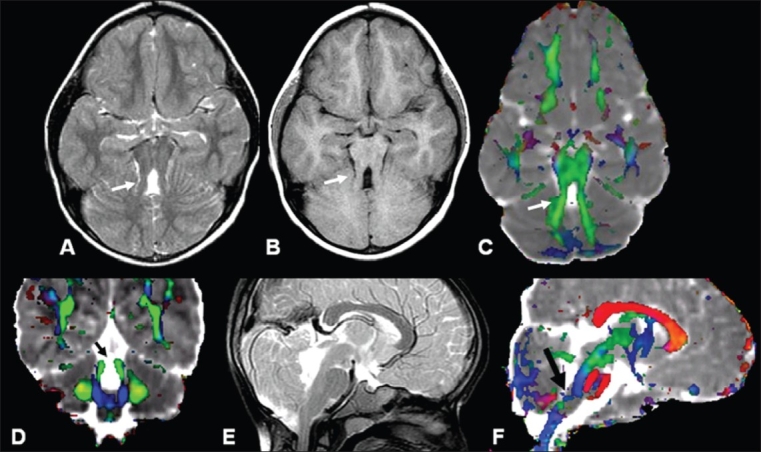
Joubert syndrome in a 2½-year-old girl with delayed development and hypotonia. Axial T2W (A) and T1W (B) MRI images show a ‘molar tooth’ appearance of the superior cerebellar peduncle (SCP) (arrow). Axial color-coded FA fused with MD map (C) shows uncrossed SCP fibers (arrow) that are clearer on the coronal image (arrow) (D). The sagittal T2W image (E) shows no obvious abnormality in the CST; however, the color-coded FA fused with MD map (F) shows thinning of the CST (arrow) at the level of the medulla
